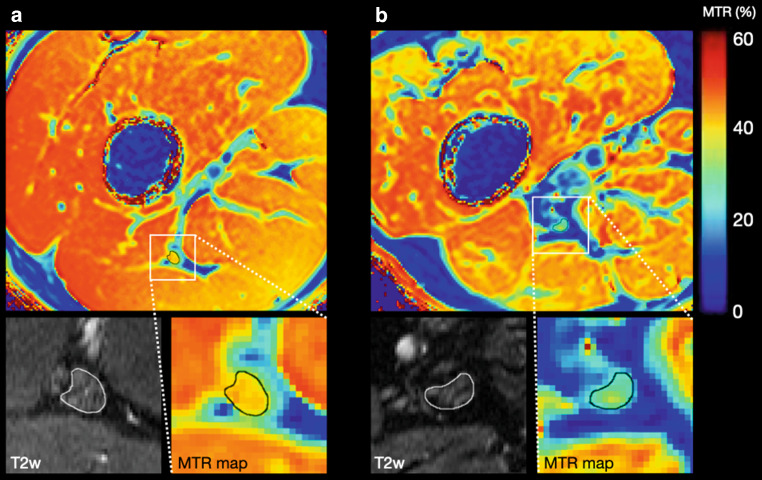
Representative magnetization transfer ratio (MTR) pseudo-colorized (%) maps of a 28-year-old (a) and a 77-year-old (b) healthy male volunteer. Boxes below show the zoomed sciatic nerve in T2-weighted (T2w) images and MTR maps. Note the decrease in MTR in the older subject compared to the younger individual
